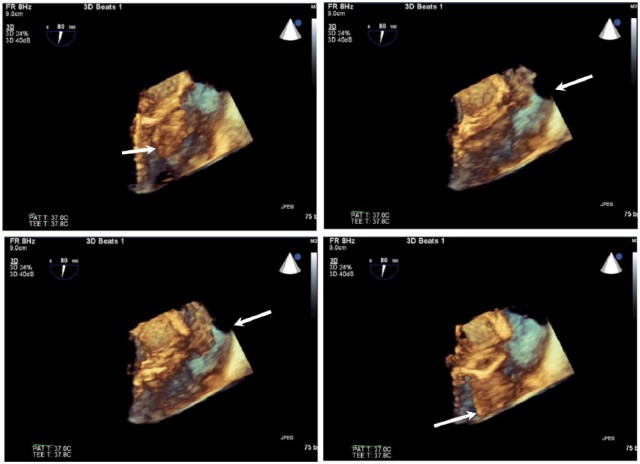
Three-dimensional transesophageal echocardiogram view of the RVOT showing pulmonic valve and hypermobile structure characteristic of vegetation from endocarditis.
